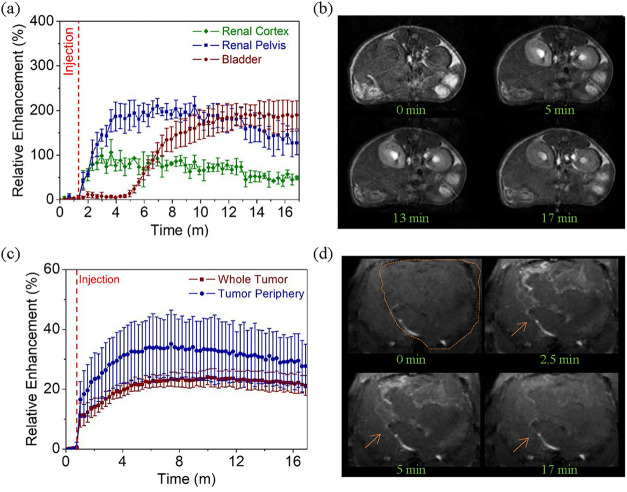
(a) *In vivo* pharmacokinetics (DCE-MRI) of renal
